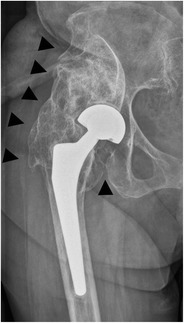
AP radiograph. Cementless bipolar hemiarthroplasty. Extensive heterotopic bone formation (*arrowheads*), bridging from femur to pelvis, restricting abduction
